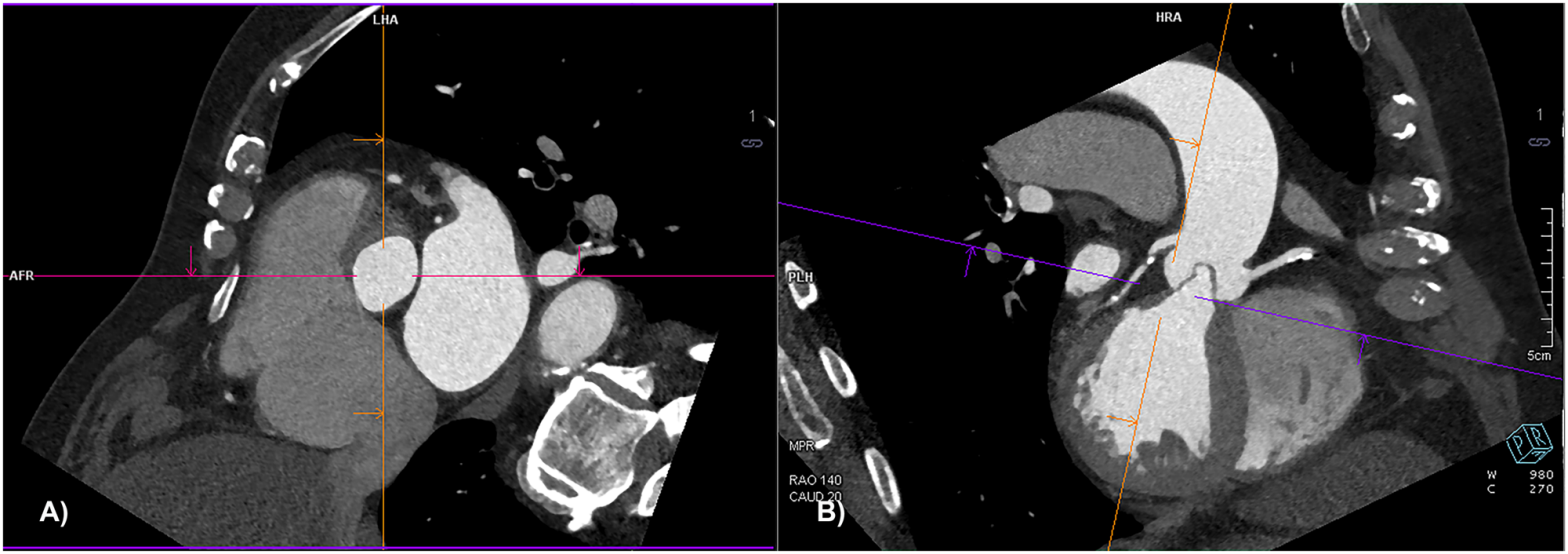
CT TAVI planning, Syngo.via^™^ VB10A Siemens, Siemens Healthcare GmbH, Forchheim Germany. (A) The oblique transversal plane crossing the most basal attachments of the aortic valve leaflets. (B) The oblique sagittal (coronal) plane of the aortic valve showing leaflets.
